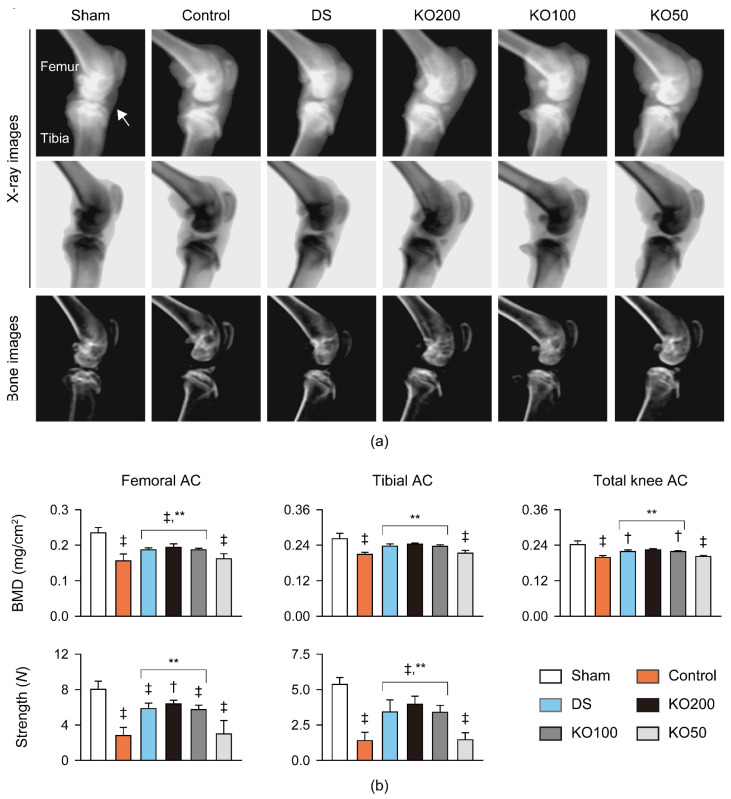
Bone mineral density (BMD) and compressive strength in vivo. (a) Representative images for joint tissues in live dual-energy X-ray absorptiometry. An arrow indicates joint capsule. (b) BMD and compressive strength in the femoral and tibial articular cartilage (AC). Values are expressed as the means ± SDs in eight sample sizes. ‡: *p* < 0.01 and †: *p* < 0.05, compared to the sham; : *p* < 0.01, compared to the control.
